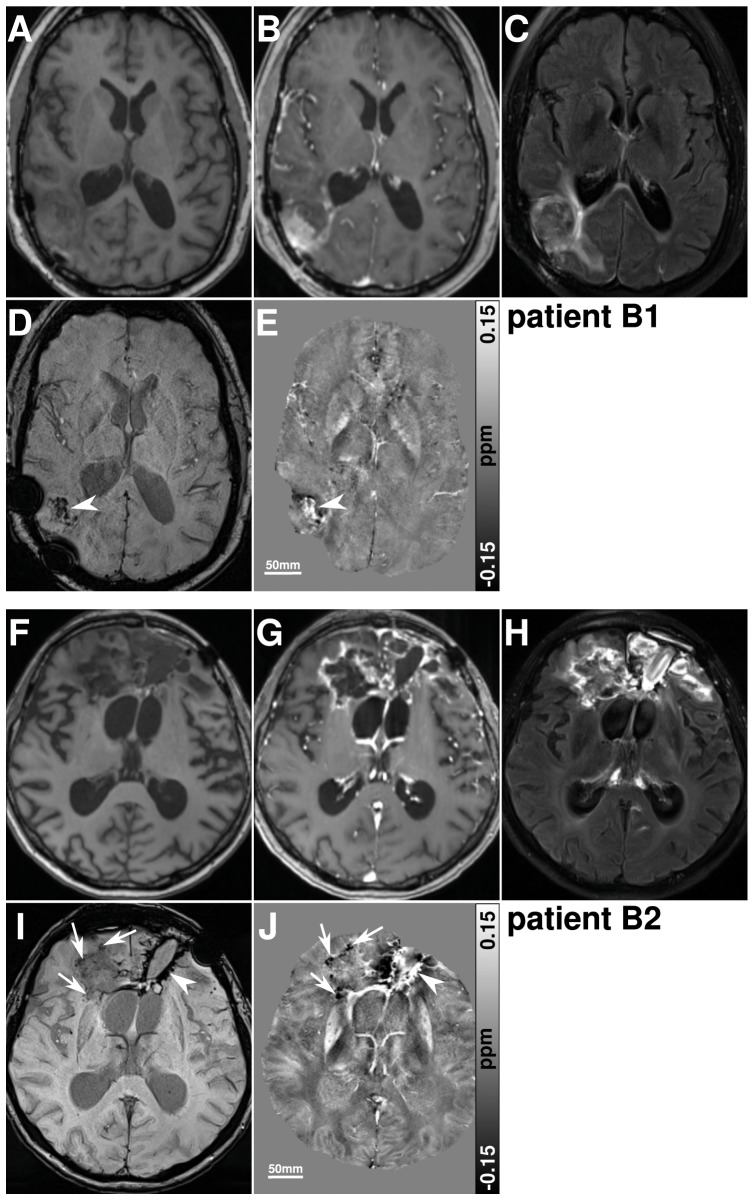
Images of two patients who were treated with bevacizumab after completion of radiochemotherapy. Images of a 42 year old man with a glioblastoma in the right occipital lobe (patient B1), who was treated with 12 cycles of bevacizumab after completion of radiochemotherapy are presented in the upper part (a–e). The lower part (f–j) reveals images of a 46 year old man with a glioblastoma in the frontal lobe (patient B2), who was treated with 5 cycles of bevacizumab. T1-weighted images before (a,f) and after contrast agent administration (b,g), FLAIR images (c,h), SW images (d,i) and susceptibility maps (e,j) are presented for each patient. The patient in the upper part represents SBS that only correlate with hyperintense areas on the susceptibility maps (arrow heads), whereas the patient in the lower part reveals additional calcifications indicated by hypointense correlates of SBS on susceptibility maps (arrows).
